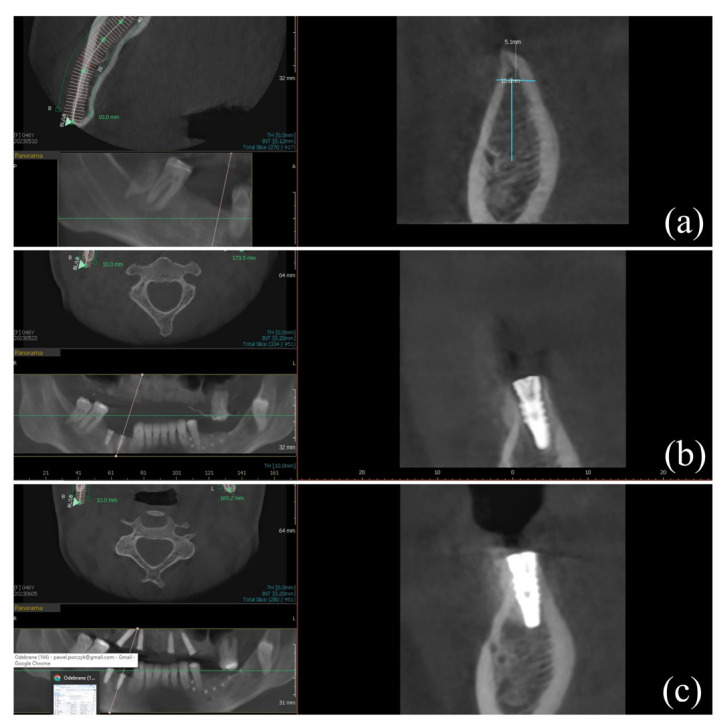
Radiographic evaluation of the clinical case shown in Figure 4, demonstrating the treatment site before and after minimally invasive bone regeneration. (a) Before (b) after surgery (c) 3 months after surgery. Colored lines are used only as visual reference markers and do not represent additional quantitative measurements.
